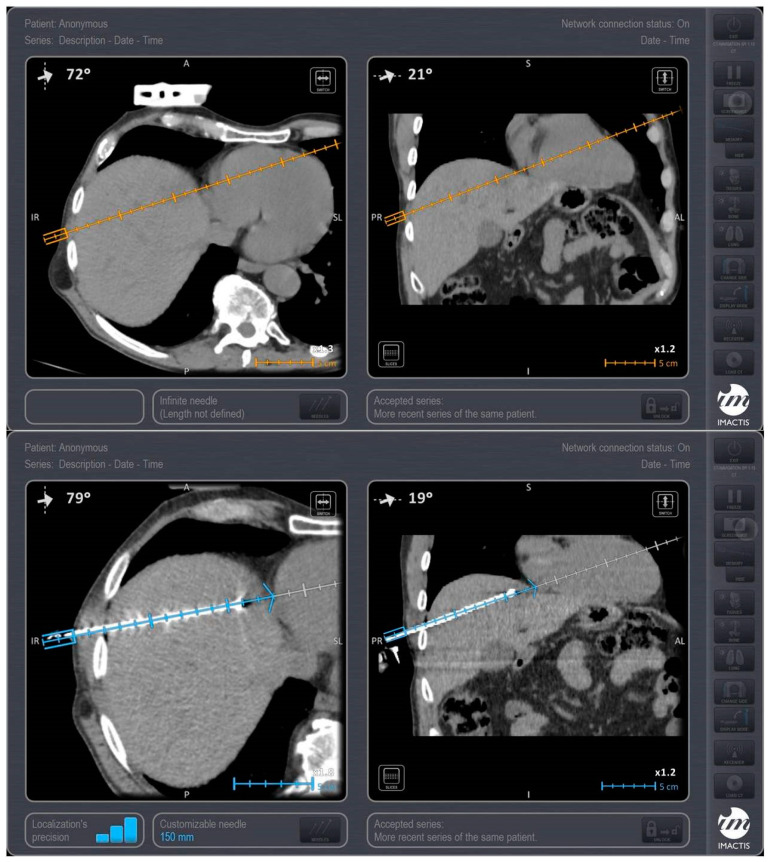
A 65-year-old male patient with colorectal cancer located at left colon with 2 synchronous metastatic lesions at hepatic dome (segment VIII). Stereotactic CT-guided ablation post systemic chemotherapy, and prior to colon resection was performed with the patient in supine position, through the anterolateral approach, under iv analgesia. Upper row: Planning scan for designing the trajectory of approach (orange line) using the IMACTIS CT navigation system. Lower row: Control scan evaluating the antenna’s position in the target lesion using the IMACTIS CT navigation system.
